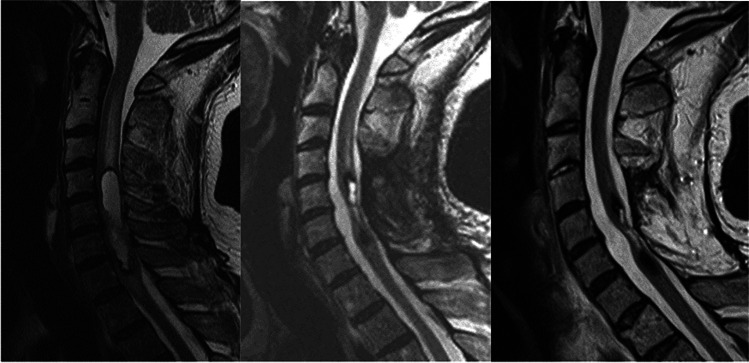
Intramedullary ependymoma. T2 weighted MRI preoperatively, 6 months and 5.5 years postoperatively
